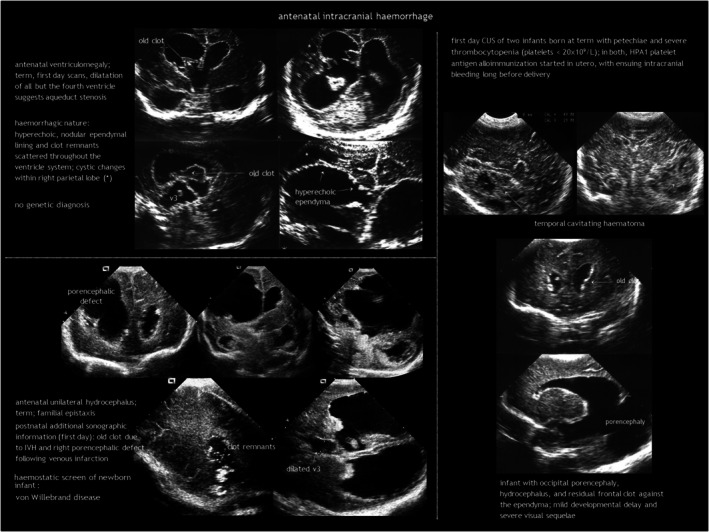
Antenatal intracranial haemorrhage. Examples of unexplained and explained cases. Abbreviations: CUS, cranial ultrasonography; HPA1, human platelet antigen 1; IVH, intraventricular haemorrhage.
